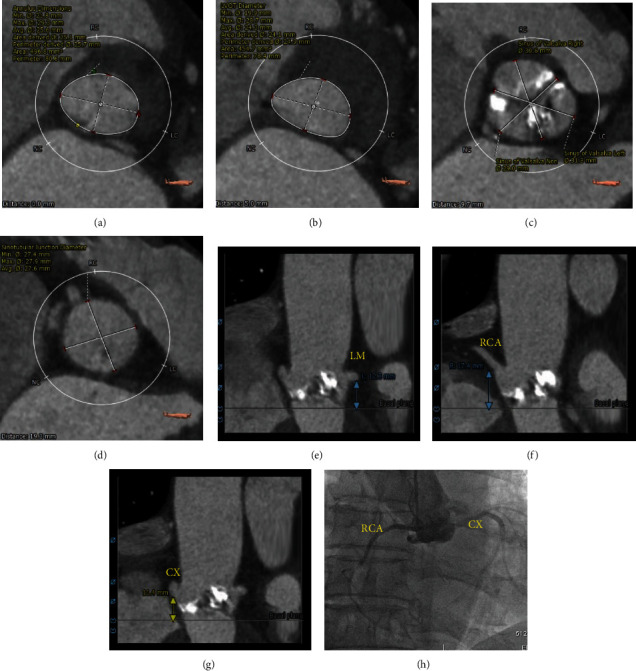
Cardiac computed tomography angiography showed severely calcified aortic leaflets with anatomical abnormalities of the right coronary system, which revealed a circumflex branch originating from the right coronary sinus, as shown by CAG.
